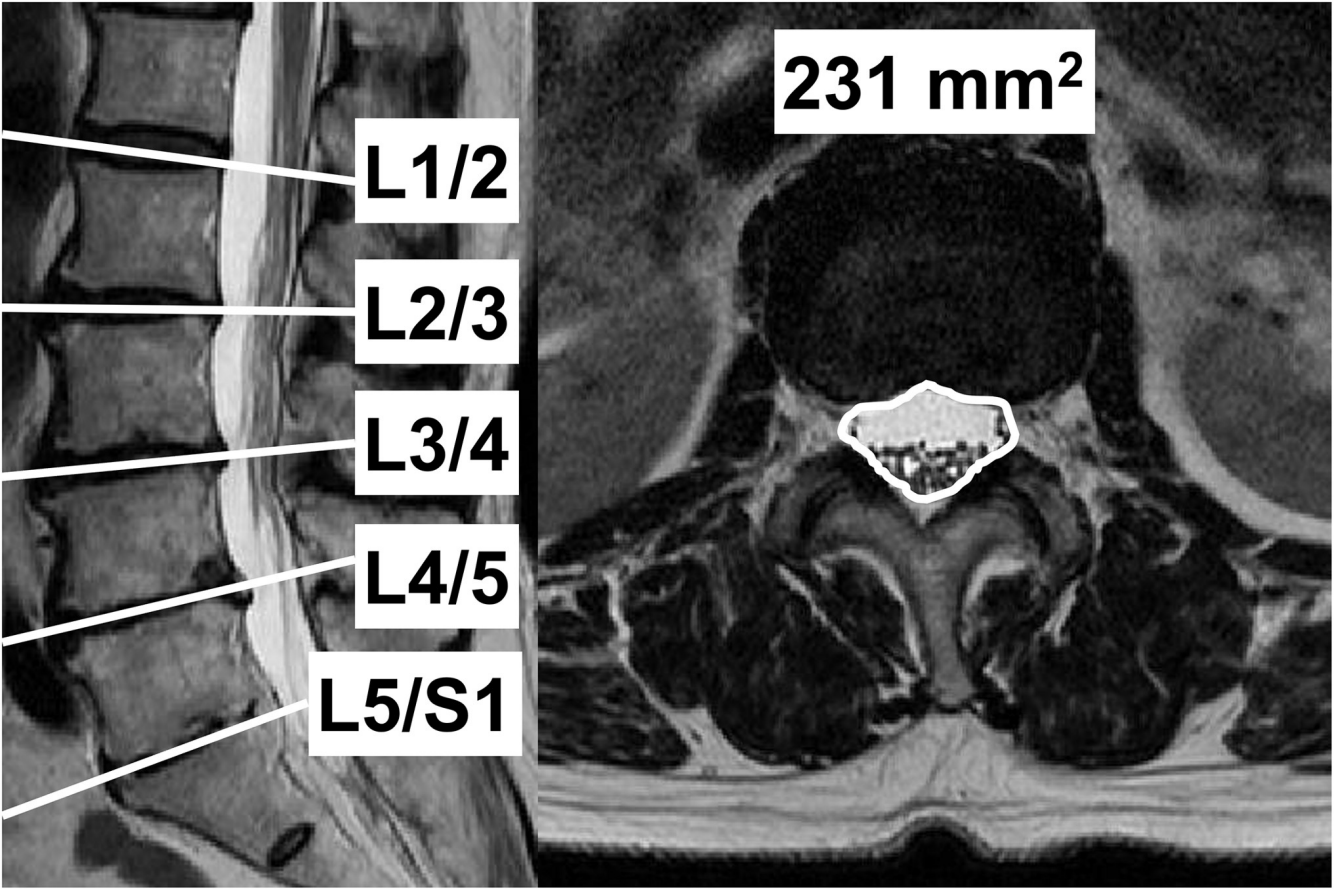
Measurements of the DCSA using MRI. Sagittal (left) and axial (right) view of the L1/2 disc level of a participant. The DCSA is surrounded by a white dotted line using axial MRI at each disc level (straight white line).
